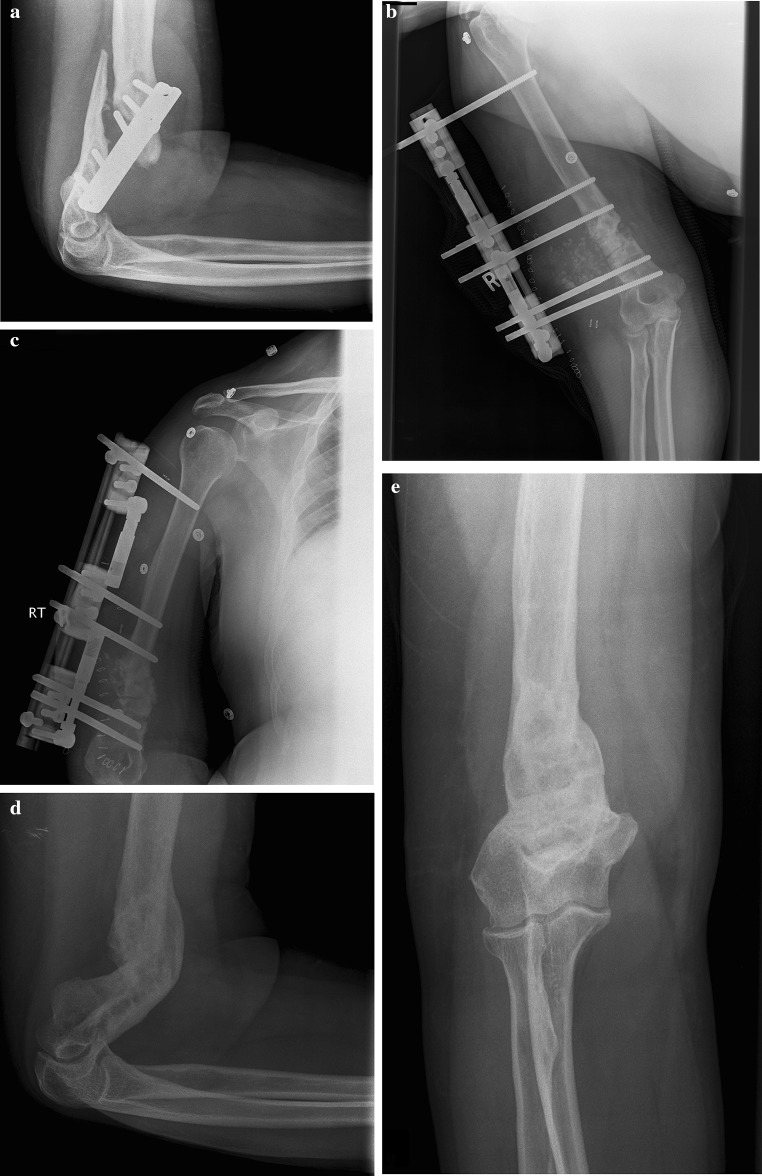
a Pre-operative radiograph of Patient 10 shows nonunion at the distal end of the humerus; b and c immediate post-operative radiographs showing the external fixator and calcium sulphate pellets in place; d and e follow-up anteroposterior and lateral radiograph shows the healed fracture
